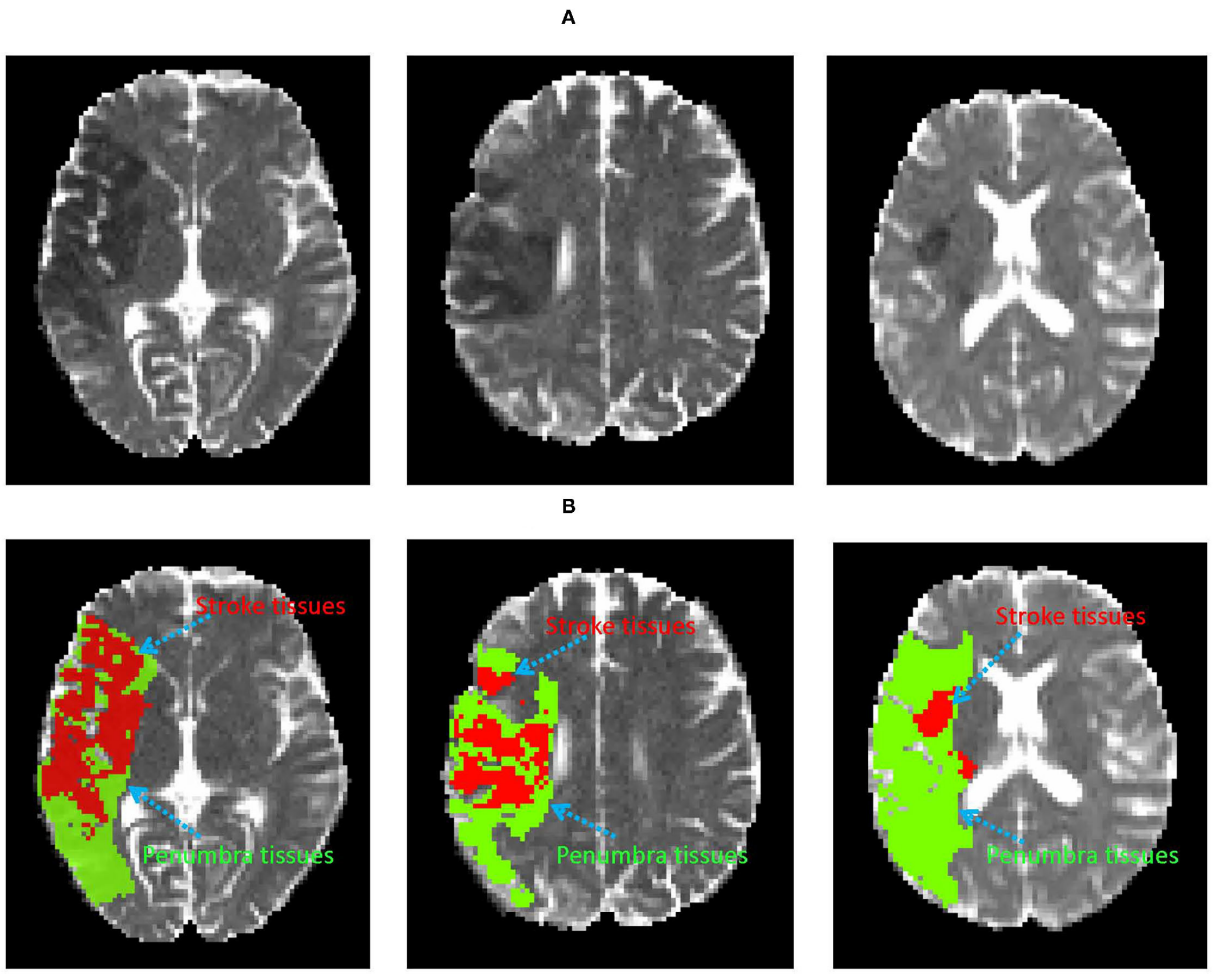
Examples of the infarct core and penumbra tissues on DWI image (A). The red and green area denote infarct core and penumbra tissues (B), respectively.
